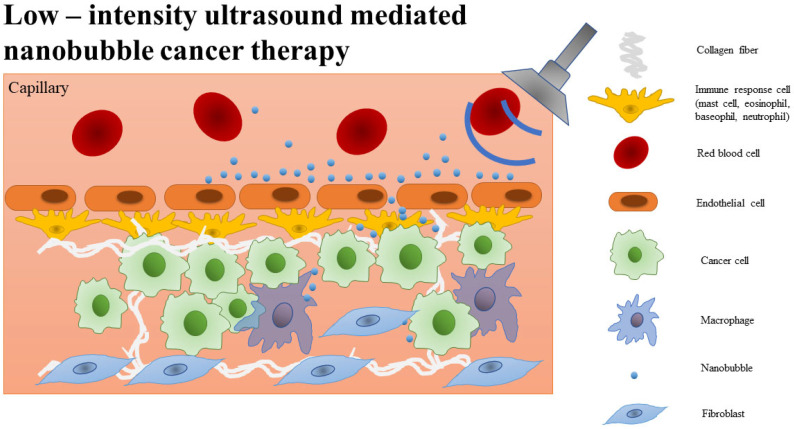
Low-intensity ultrasound-mediated nanobubble cancer therapy.
